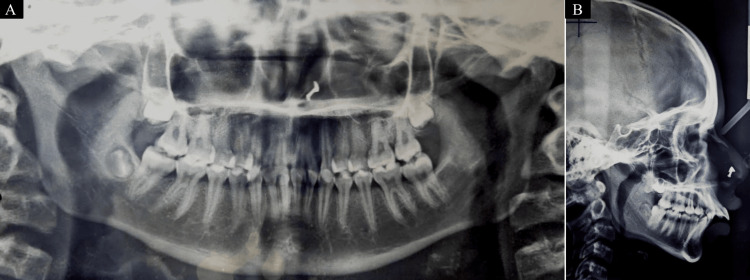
Pretreatment records (radiographs). (A) Orthopantomogram. (B) Lateral cephalogram
